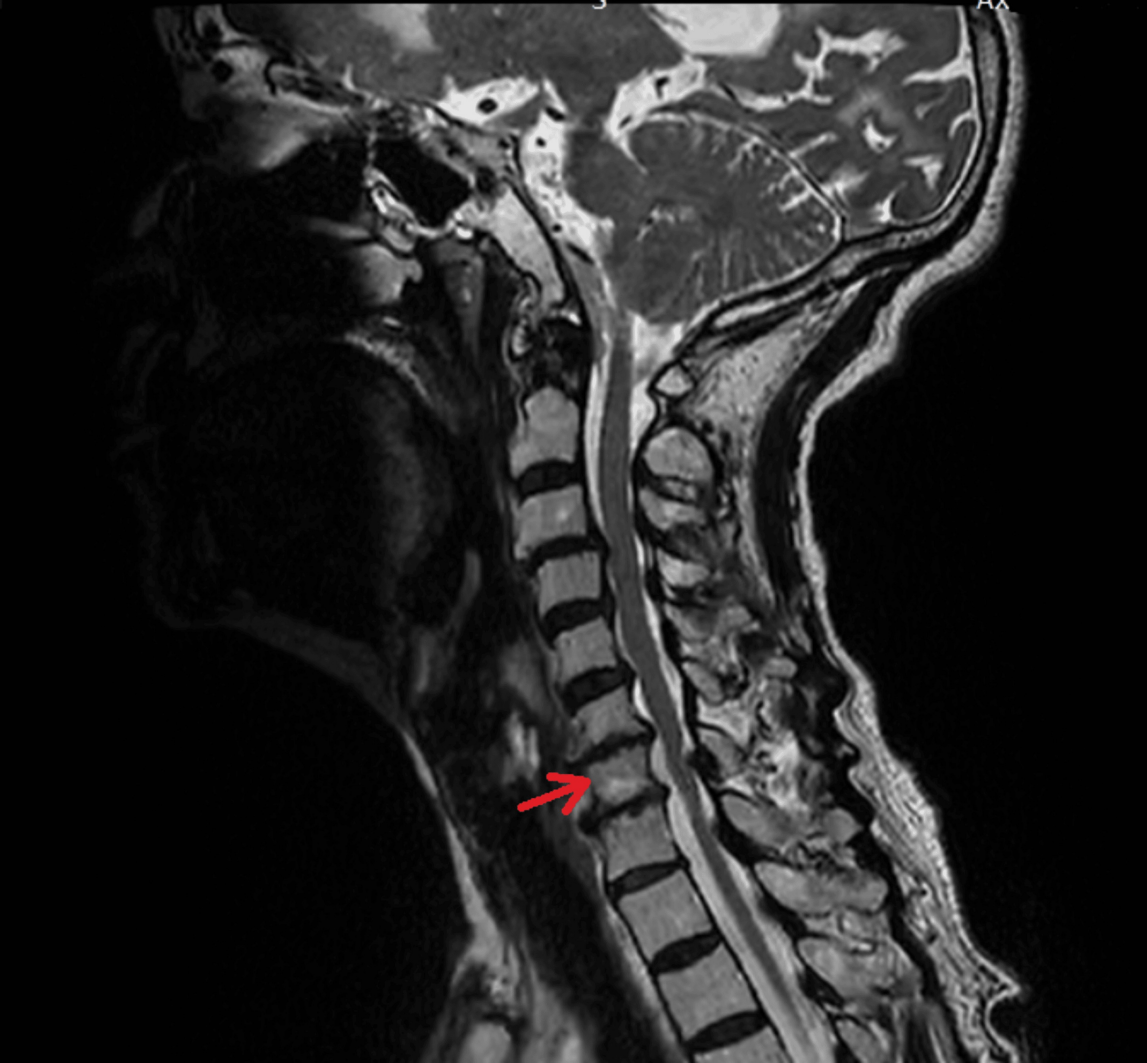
Cervical spine MRI demonstrating multilevel degenerative changes Sagittal T2-weighted MRI showing multilevel degenerative changes and mild to moderate spinal canal stenosis from C4 to C7
